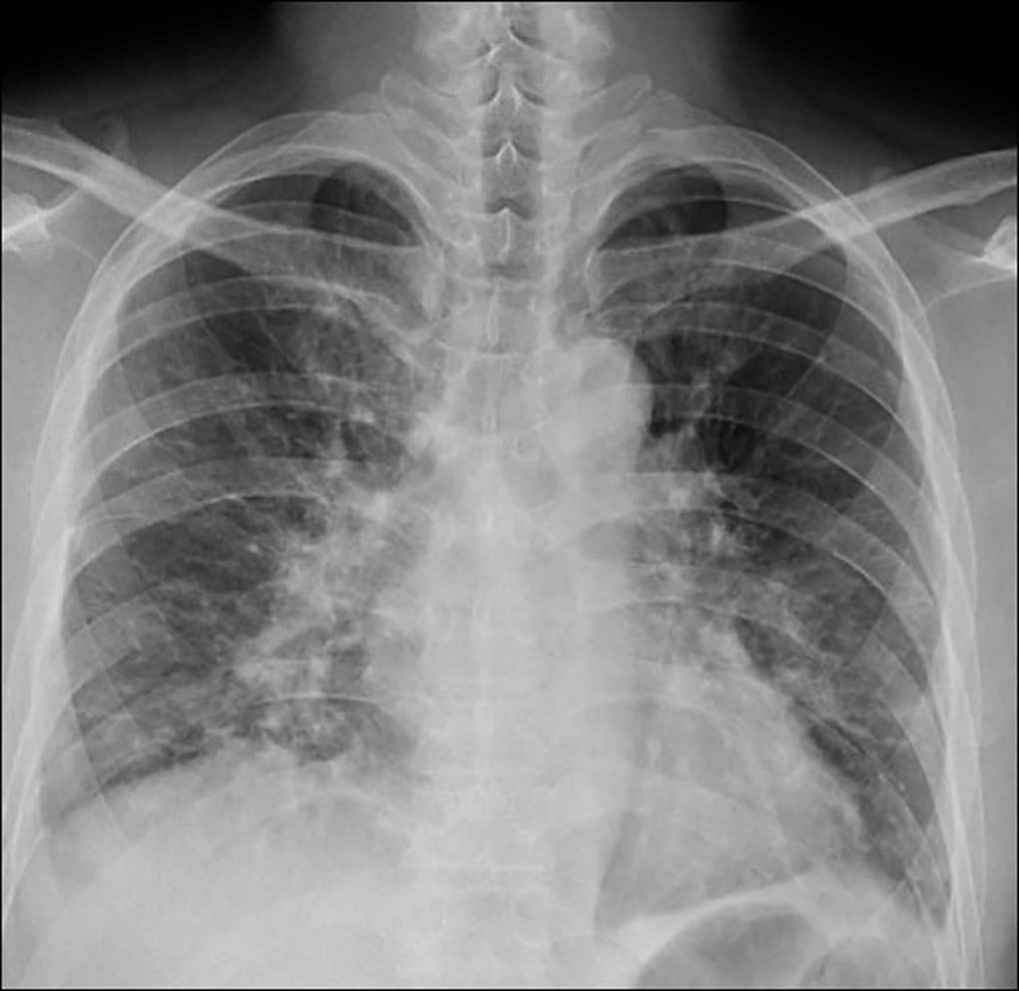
Chest radiograph showing diffuse ground-glass opacities in both lung fields.
